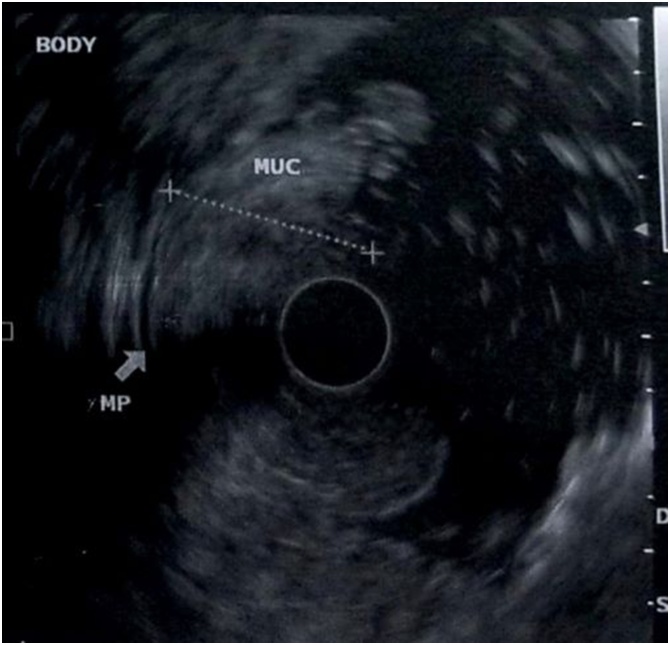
The endoscopic ultrasound shows the severe mucosal thickness.
